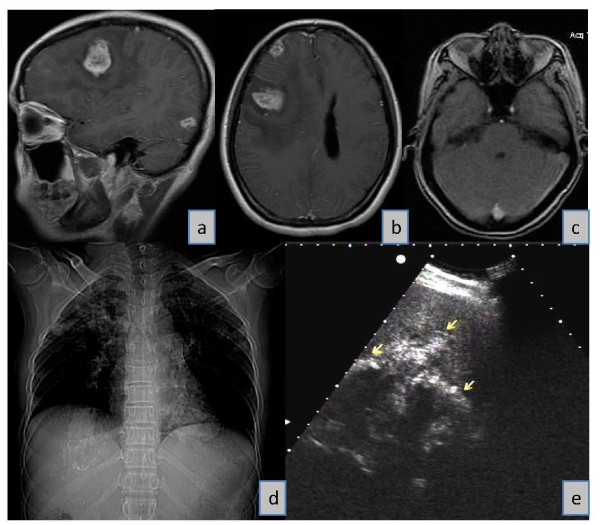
Patient diagnostic images: Magnetic Resonance Imaging (*Panels a, b, c*): *Panel a *is a sagittal T1 image of the brain showing AE lesions in the temporal (the largest lesion is 3.5 × 3 cm in size) and occipital lobes; *Panel b *is an Axial T1 image of the brain showing AE lesions in the frontal and temporal lobes; *Panel c *is an Axial T1 image of the brain (at a lower level than *Panel b*) showing a lesion in the occipital lobe; *Panel d *is a chest x-ray image showing many cotton-flower shape shadows spreading on both sides of the lungs, but mainly involving the upper and middle lung areas; *Panel e *is an abdominal ultrasound scan showing a P4 AE lesion (arrowed) in the right liver lobe.
